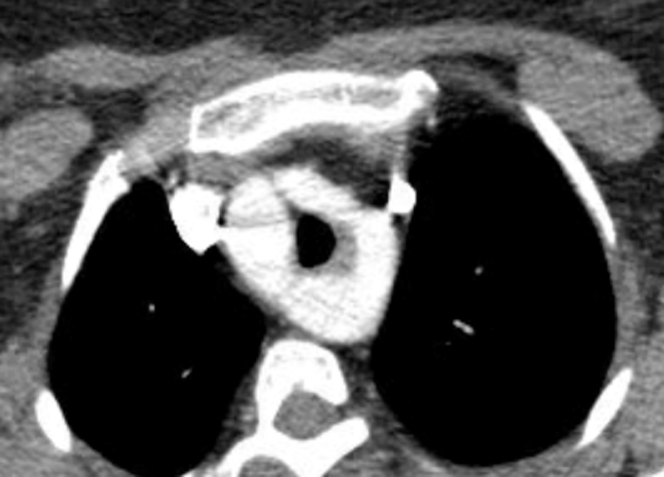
Computed Tomography–Enhanced Image of Vascular Ring Around Trachea With Persistent Left Superior Vena Cava
